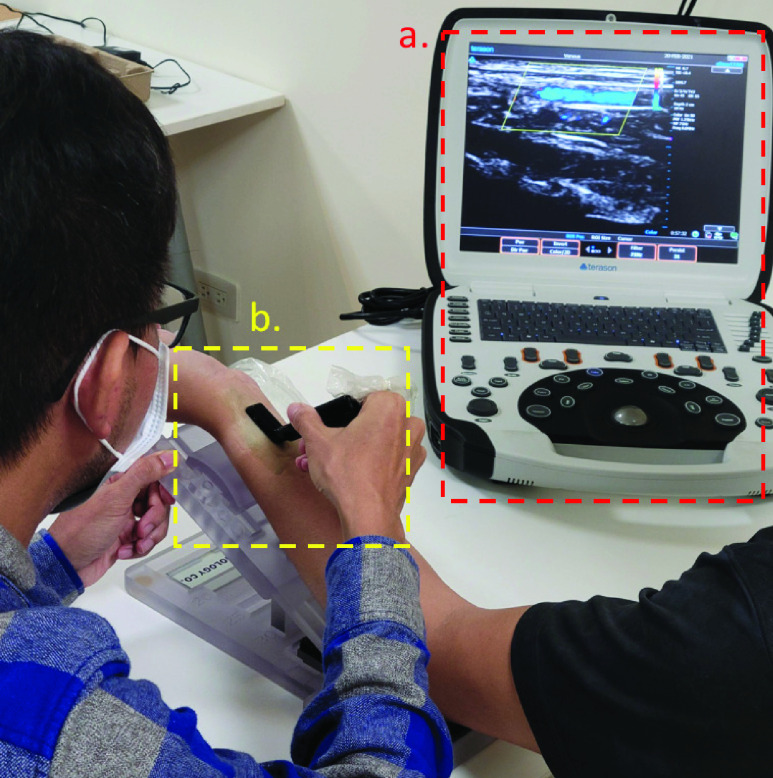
a) The portable ultrasound scanner uSmart-3300 produced by Terason. b) The L-Type ultrasound probe used to collect the ultrasound image of the participant’s radial artery.
